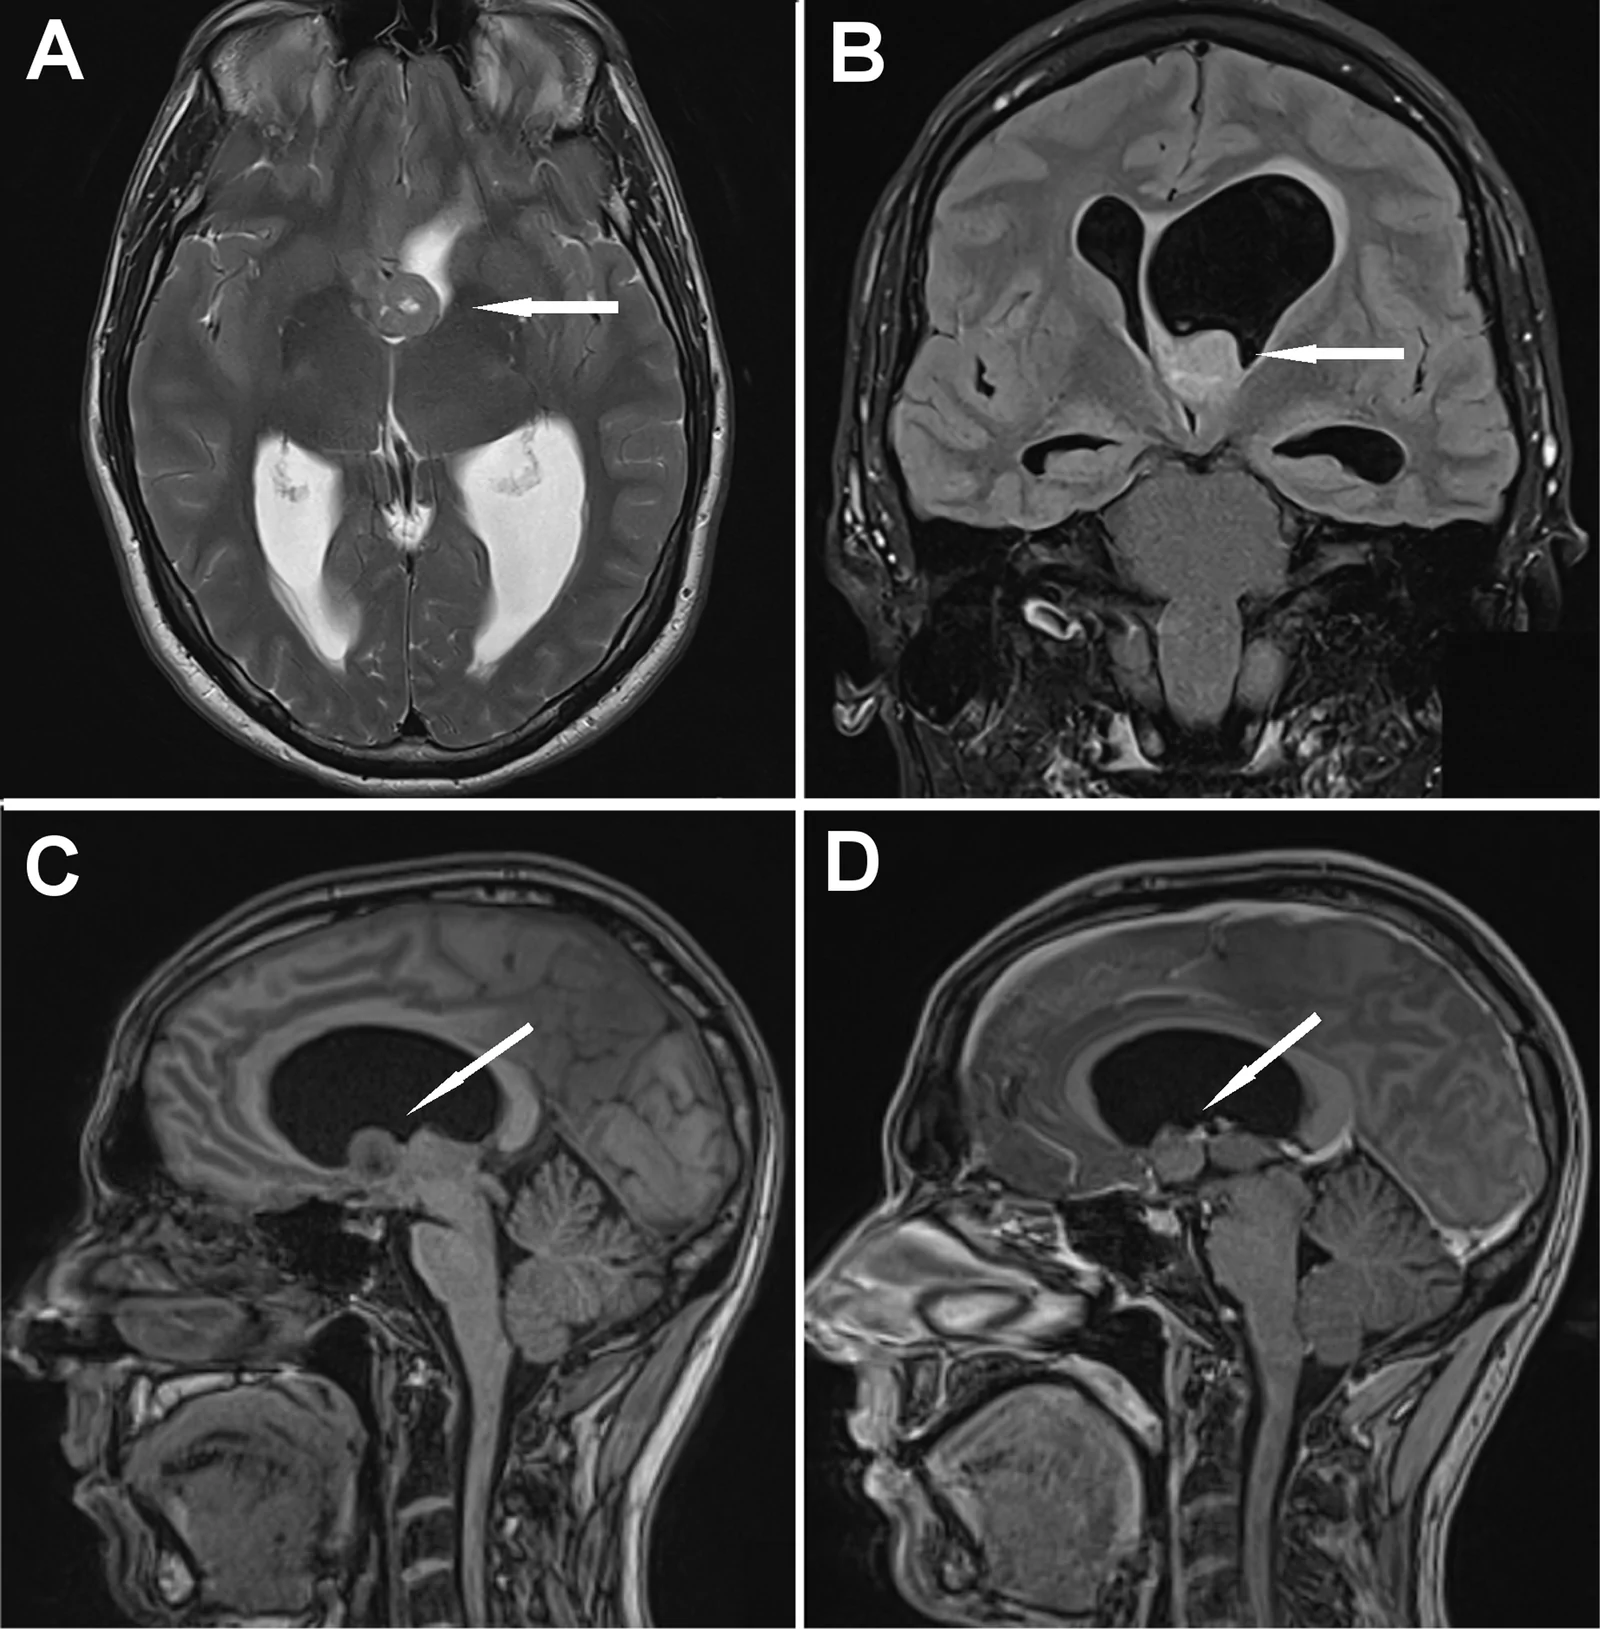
Figure 1. Neuroradiological features of this case of central neurocytoma. A: T2-weighted images, axial view. Moderately well-defined solid tumor (arrow) with small microcysts appears isointense relative to the cerebral cortex. B: FLAIR images in the coronal view. The tumor (arrow) shows hyperintensity compared to the brain. C: Non-contrast T1-weighted images in the sagittal view. The tumor (arrow) appears isointense compared to the gray matter. D: Contrast-enhanced T1-weighted images in the sagittal view. The tumor (arrow) shows mild-to-moderate, slightly heterogeneous enhancement.
